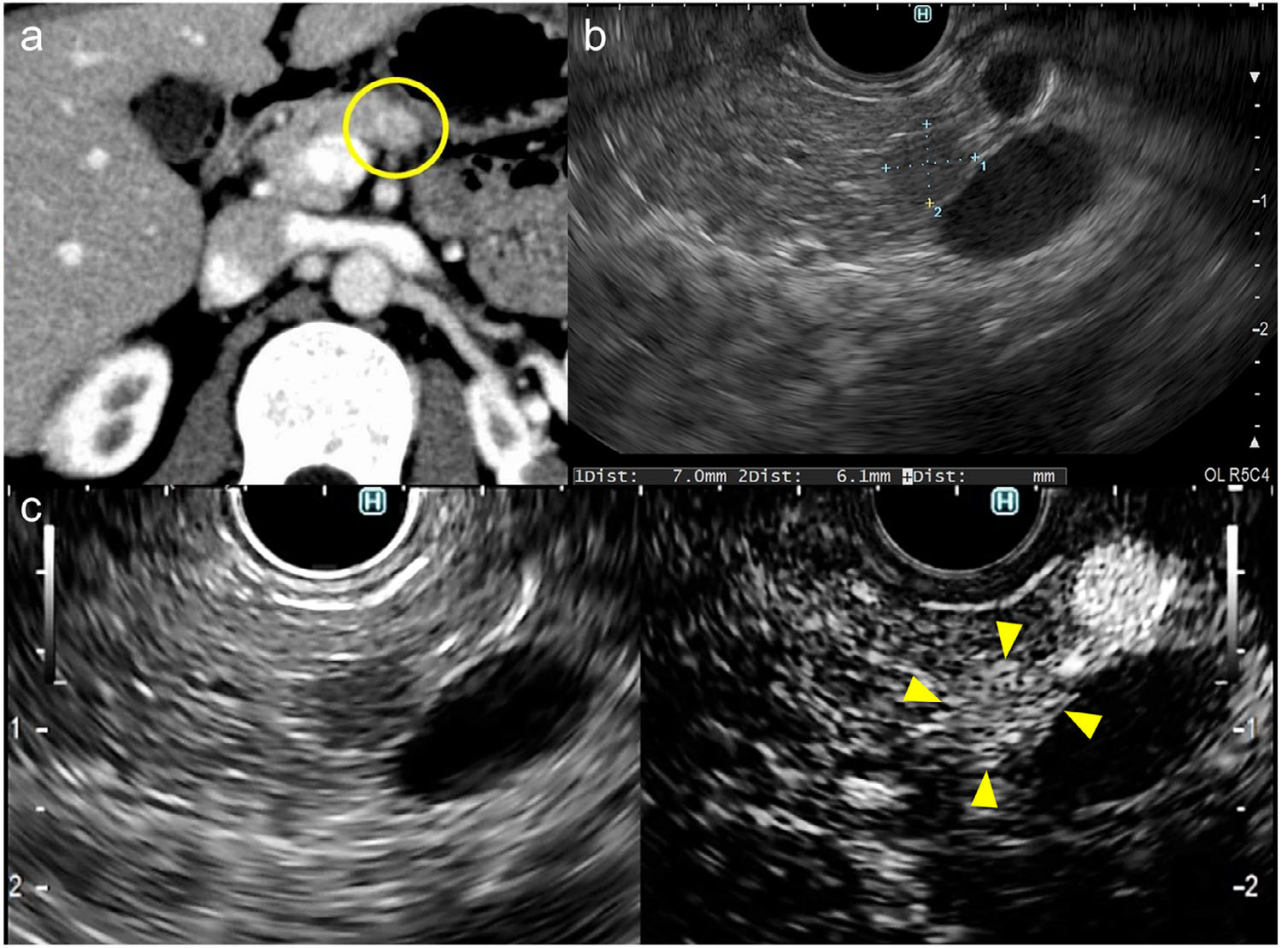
Imaging findings of the small pancreatic body lesion. (a) Contrast‐enhanced computed tomography demonstrating an early‐enhancing nodule in the pancreatic body (circled area) during the arterial phase. (b) Endoscopic ultrasonography revealing a 7 mm well‐defined, round‐to‐oval, hypoechoic mass. (c) Contrast‐enhanced endoscopic ultrasonography showing early‐phase hyperenhancement of the mass relative to the surrounding pancreatic parenchyma (arrowheads); the left panel shows the monitor image, and the right panel shows the contrast‐enhanced image.
